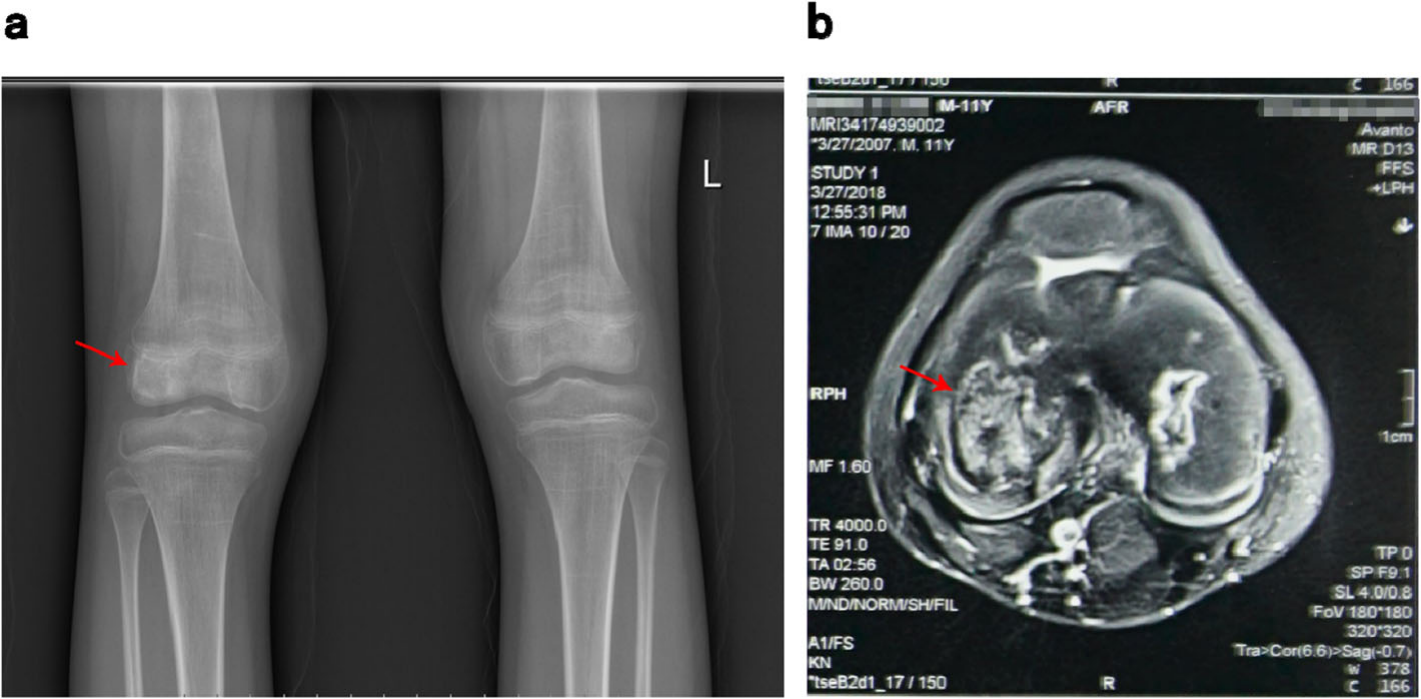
X-ray scan (a) and magnetic resonance imaging report (b) of knee joints (The arrows point out pathological structures in examinations, indicating necrosis of the right knee osteoepiphysis)
